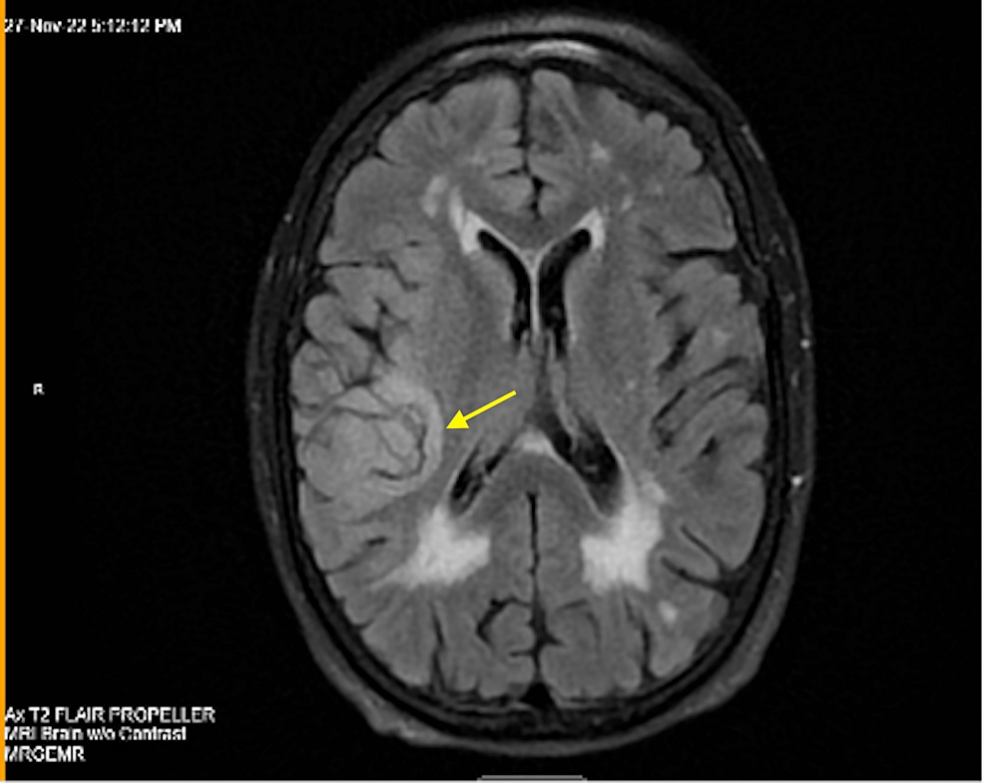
MRI of the brain without contrast revealed cerebral edema, sulcal effacement and cortical diffusion restriction within the right temporal and right posterior parietal regions.
